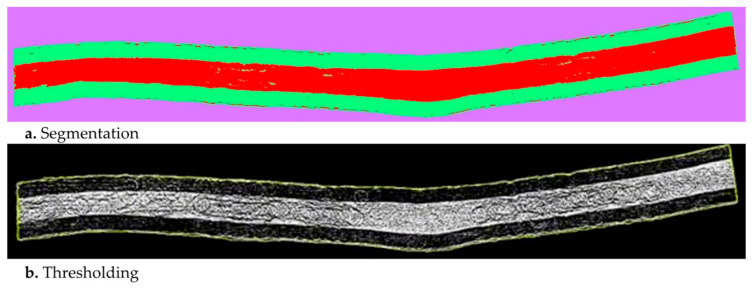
Image processing of the CT images: (a) region segmentation with Image J of prepreg sample representative slice for the (purple) background, (green) resin, and (red) fiber and (b) region of interest adapted for each slice of the prepreg sample (highlighted as yellow lines).
